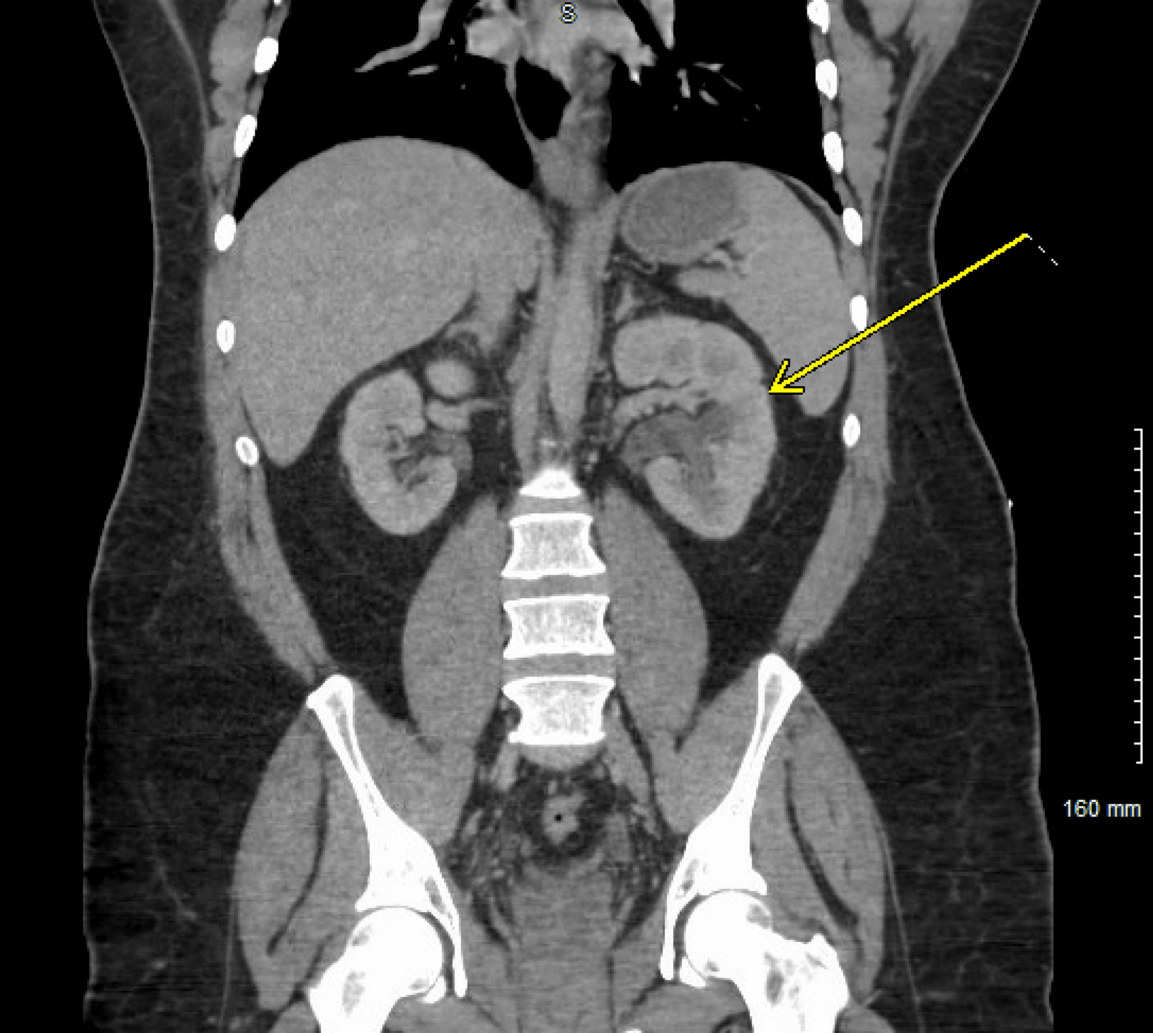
Left hydronephrosis CT scan of the abdomen and pelvis showing mild left hydronephrosis, pointed by a yellow arrow
